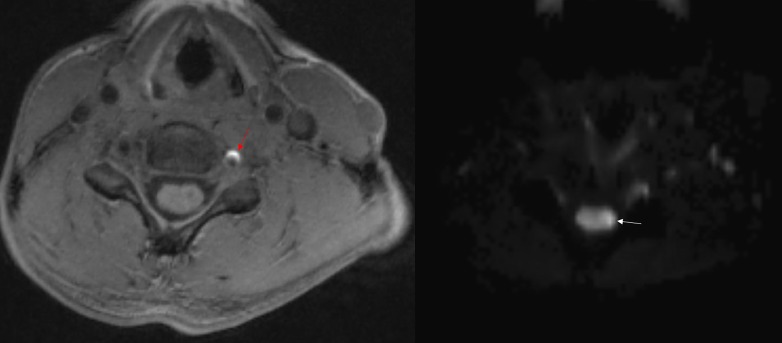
Axial T1-weighted (MPRAGE) image with fat saturation demonstrates left vertebral artery dissection with luminal narrowing and hyperintense intramural hematoma (red arrow) Axial diffusion weighted image (DTI trace) at the same level demonstrates diffusion restriction (white arrow).
